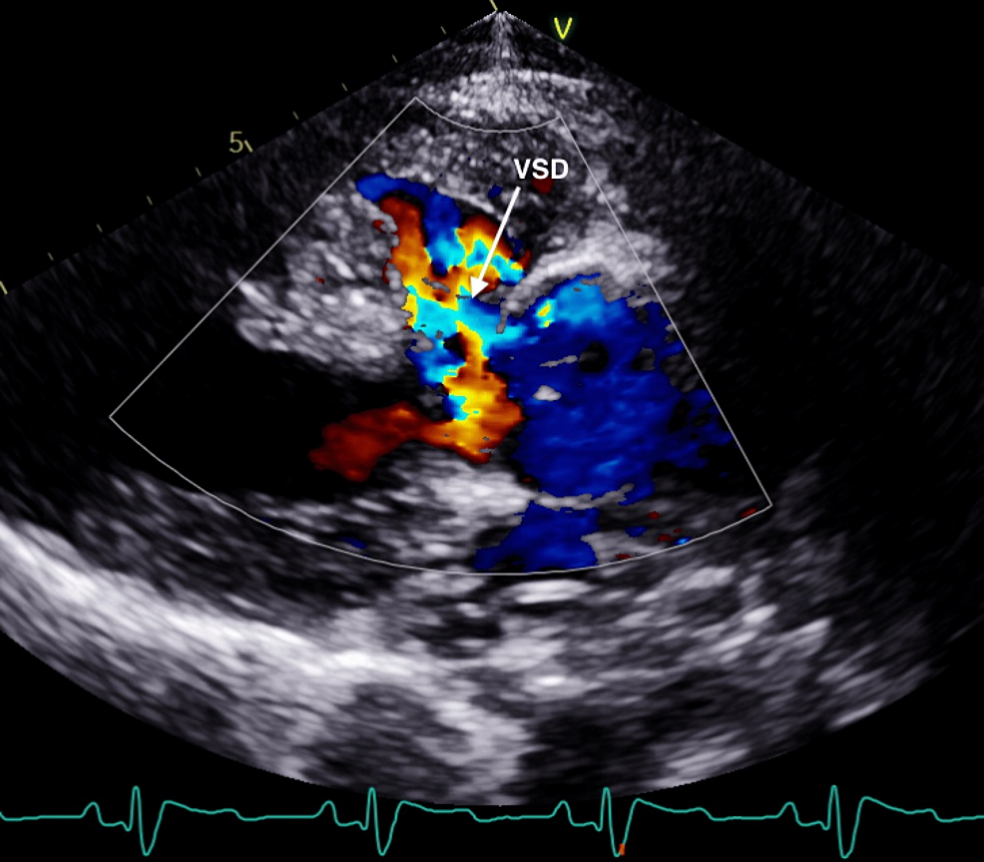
Echocardiogram with color doppler showing right-to-left shunt VSD
